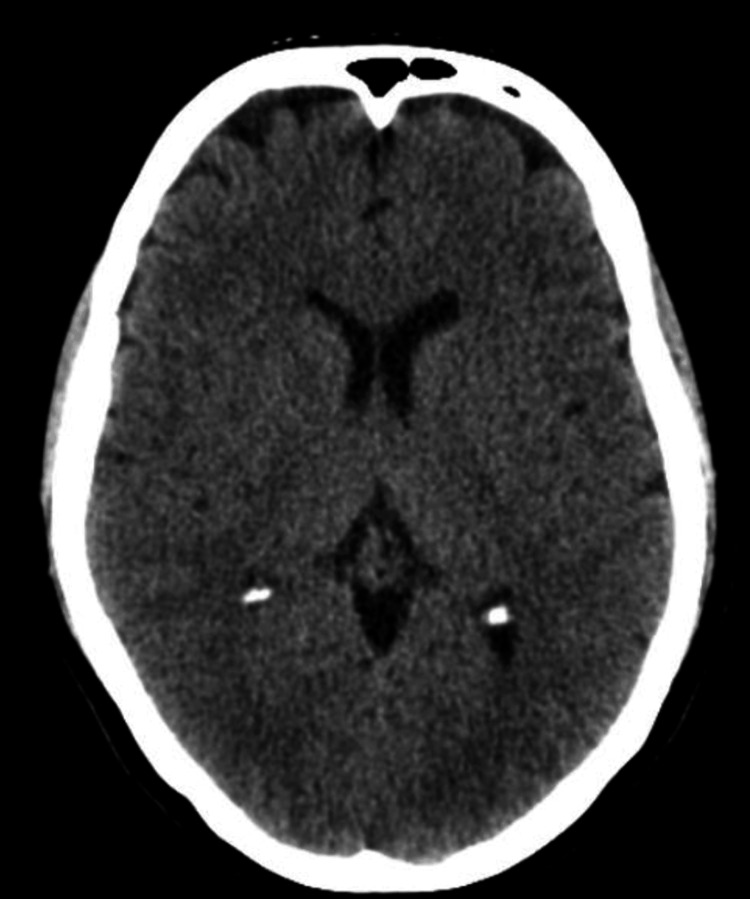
CT of the brain without contrast Initial negative brain CT showing no evidence of vessel occlusion, hemorrhage, or mass effect.
